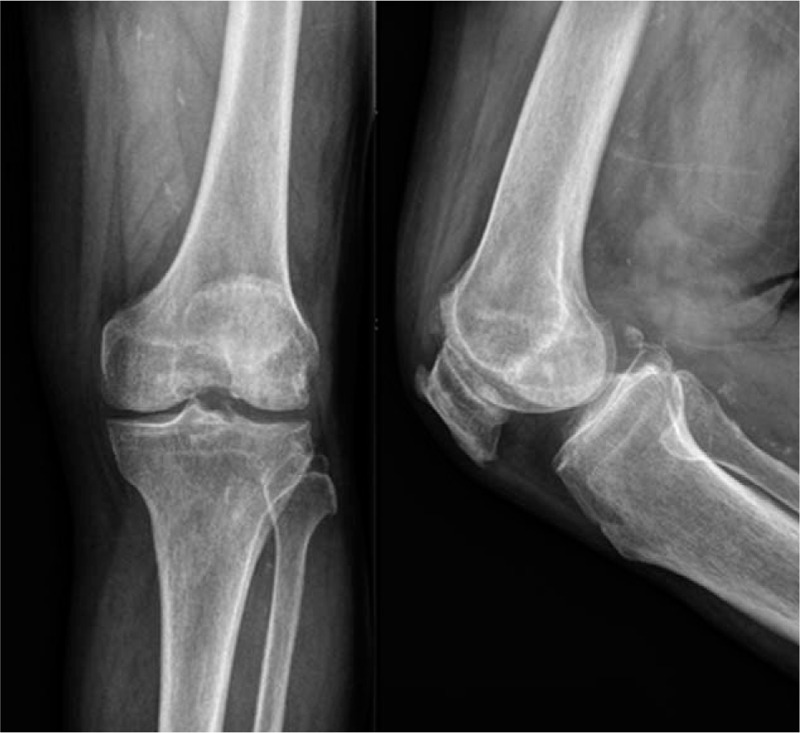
There were no signs of recurrence on outpatient follow-up X-ray until 1 year after embolization.
